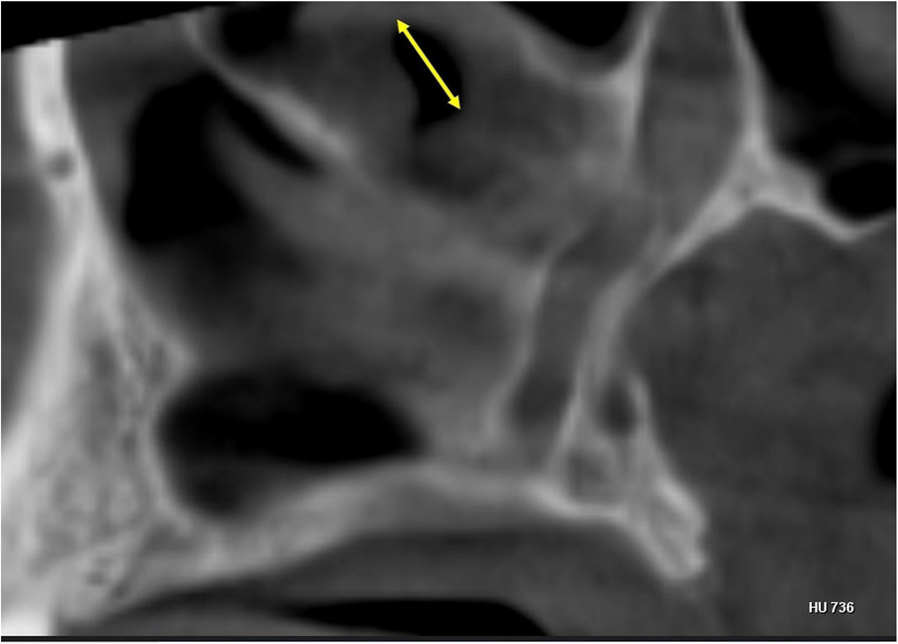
Two-sided yellow arrow depicting the maximal length of the AMO located on the lateral nasal wall observed in the sagittal CBCT section
